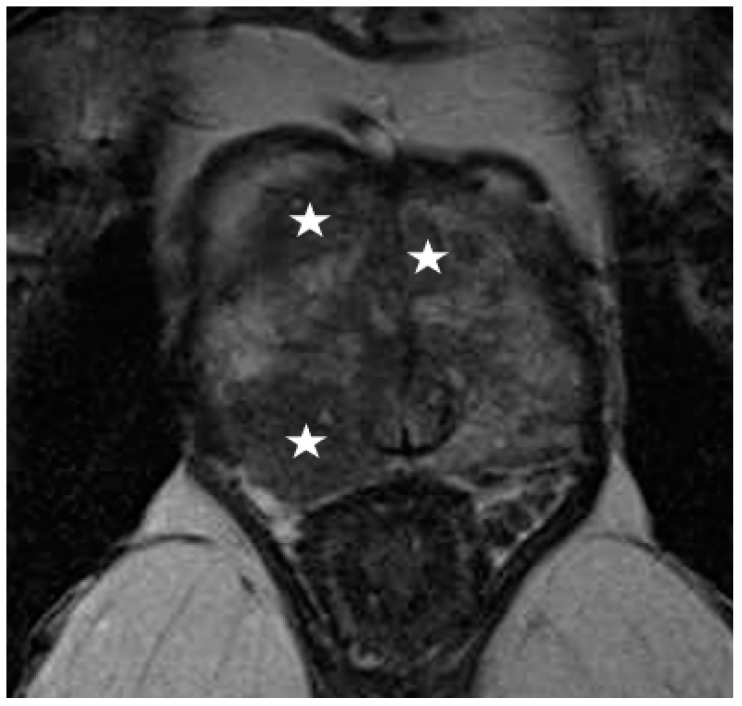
Multifocal prostate cancer seen as hypointense lesions on T2-weighted imaging (star), obscuring the boundaries between peripheral and transition zone, making zonal segmentation challenging.
